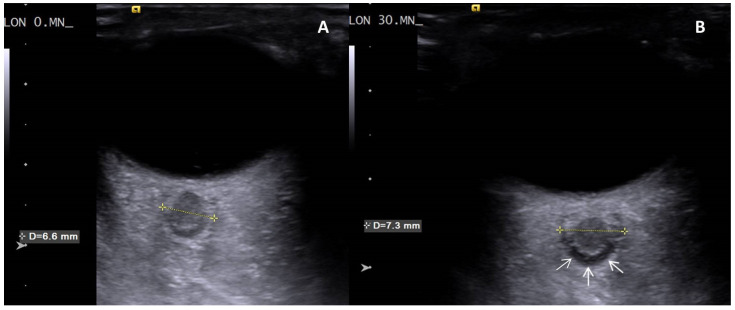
Transorbital ultrasound visualization of the axial plane of the left eye. The optic nerve appears hypoechoic and is surrounded by the hyperechoic optic nerve sheath. (A) Optic nerve sheath in a volunteer with normal intracranial pressure at T0 showing no visible subarachnoid space around the optic nerve. (B) Optic nerve sheath in the same volunteer at the 30th minute of cervical hyperextension position demonstrates a significant subarachnoid space around the optic nerve (white arrows), indicating elevated intracranial pressure.
